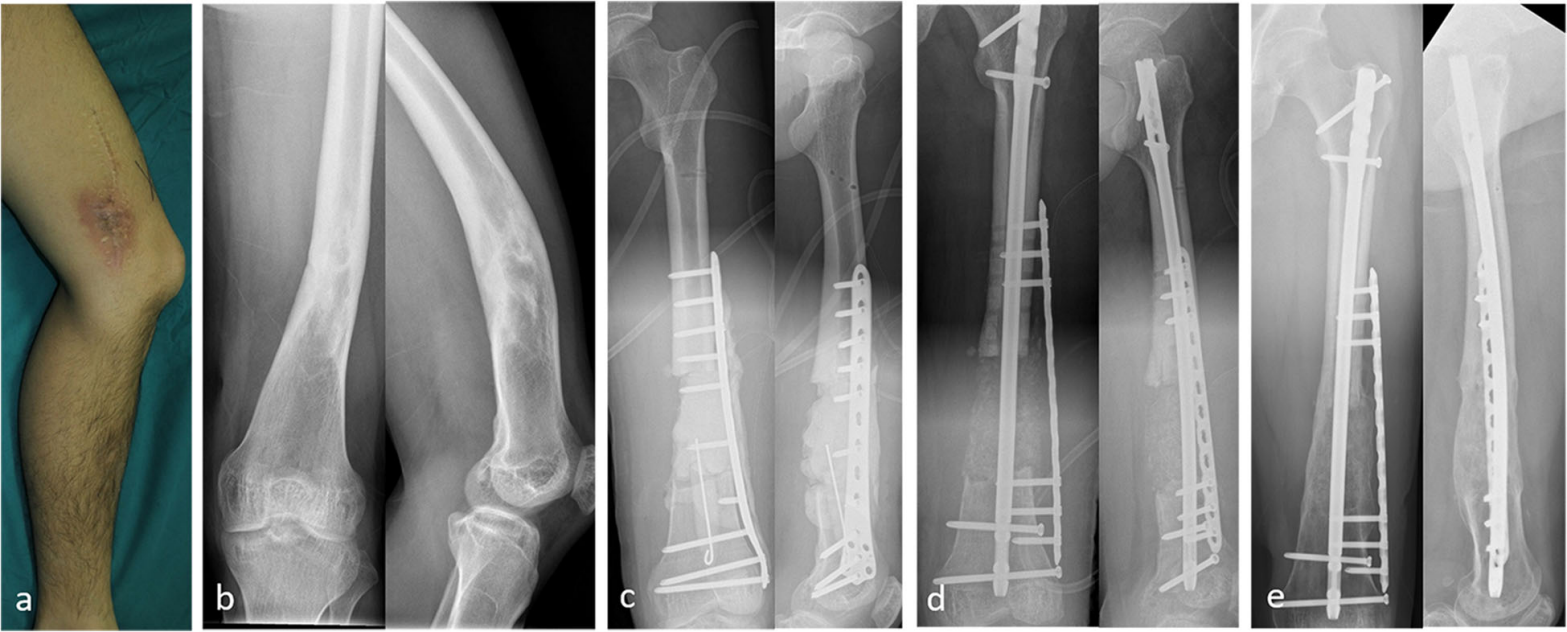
Thirty-year-old male. Bone infection of the right femur for 18 years. The culture results showed *Staphylococcus aureus*. a Preoperative photo. b Preoperative X-rays. c X-rays after the first stage. d X-rays after the second stage. e Bone union after 24 months
